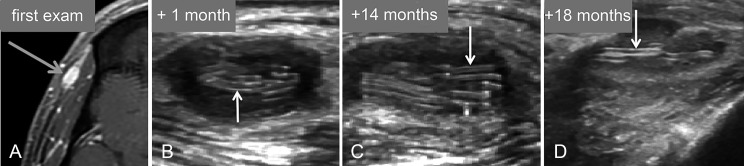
Temporal region examinations previously performed at other institutions. (A) T1-weighted MRI, performed after intravenous administration of contrast agent, shows a small hypodermal formation with a high signal corresponding to vascularized tissue (arrow). (B) to (D) Ultrasounds respectively performed 1 month, 14 months, and 18 months later all show the overall hypoechoic appearance of the lesions, containing curious fine echogenic structures in the shape of a ‘rail’ (arrows).
